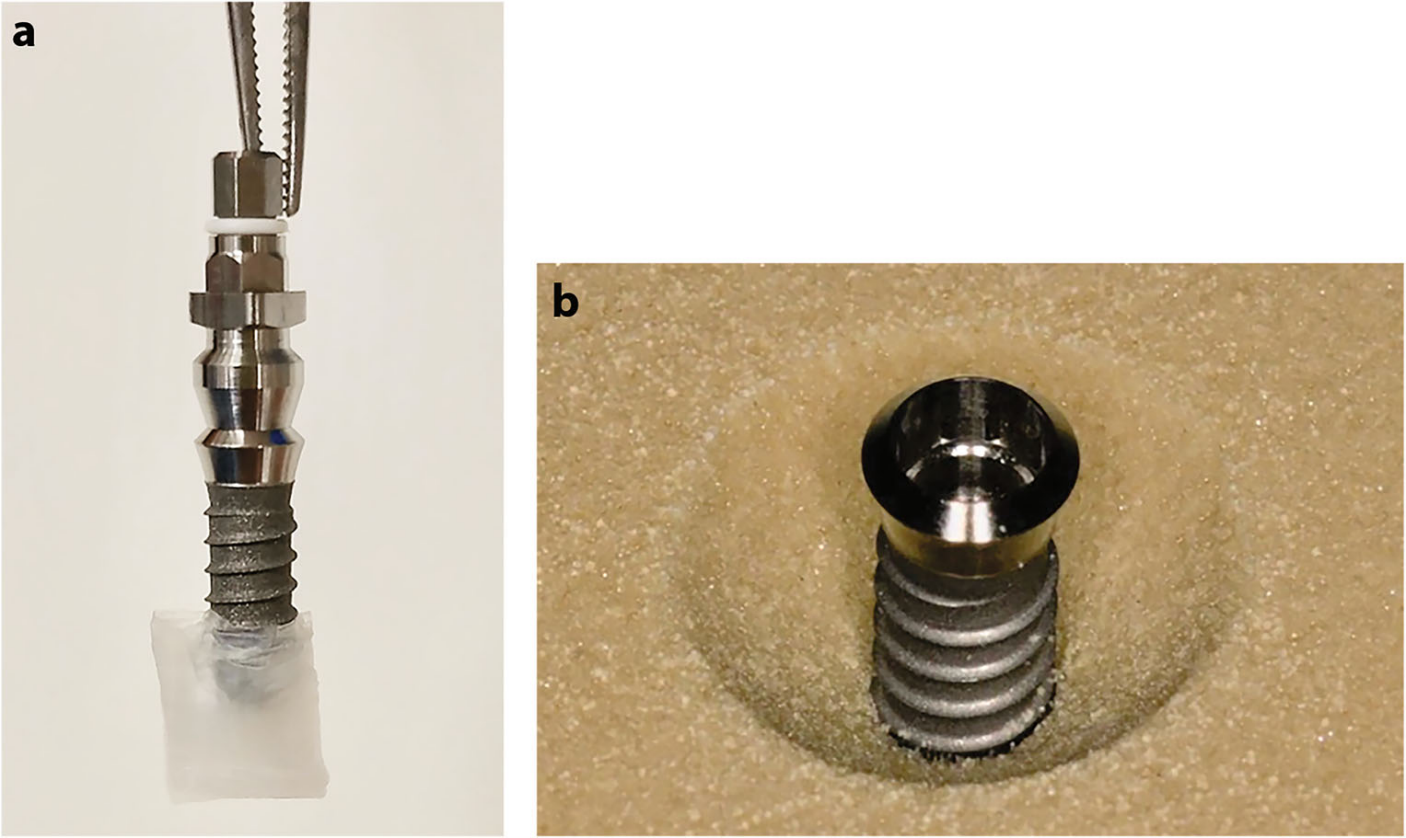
a Parafilm wax coating of implant’s apical 4mm. b Peri-implantitis model of Sawbone defect
